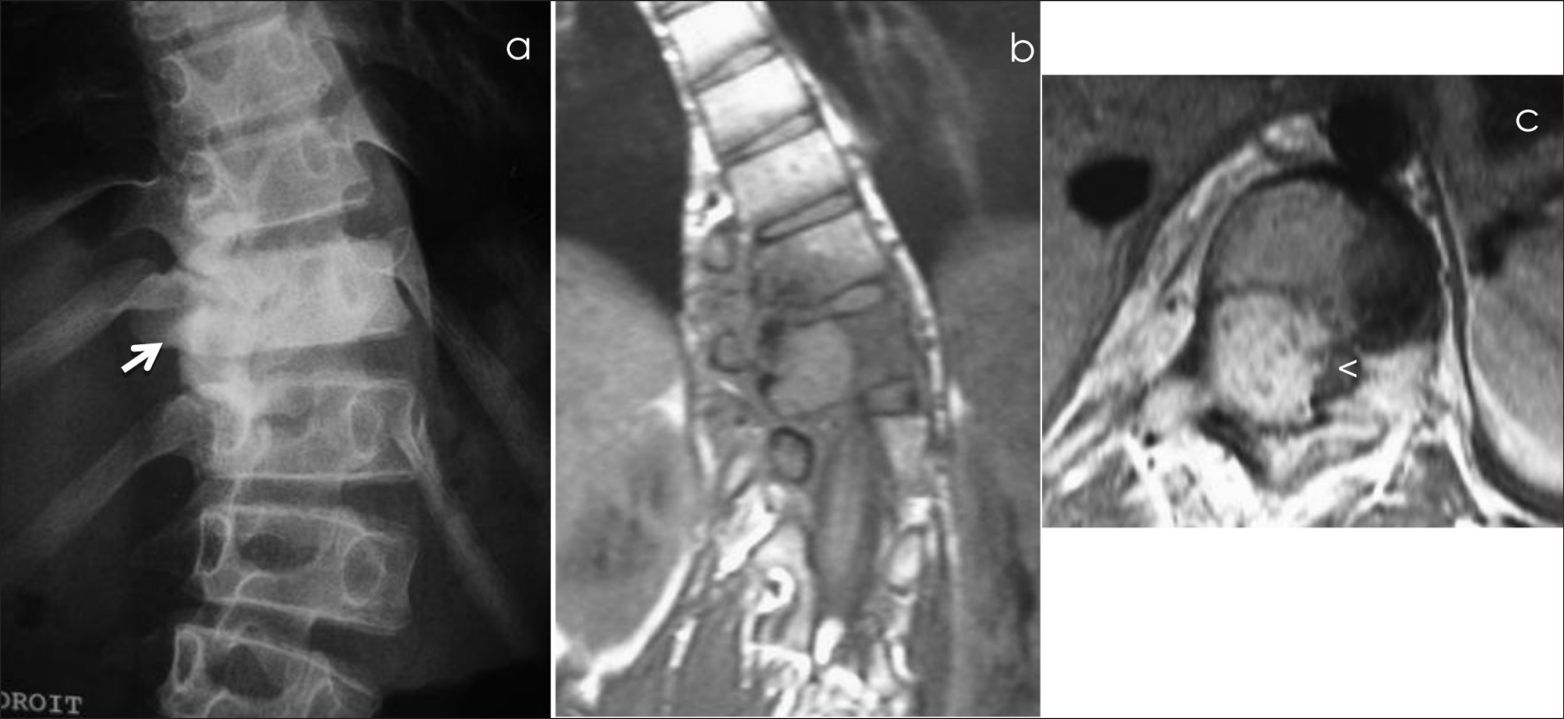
Osteoblastoma of T11. Radiograph (a) shows scoliosis and focal sclerosis of T11 (arrow). Coronal T1-WI (b) and axial T1-WI after gadolinium contrast administration (c) shows a lesion of the neural arch of T11, intralesional calcification and adjacent bone marrow edema of the vertebral bodies of T10 and T11. Note the compression of spinal cord (arrowhead).
